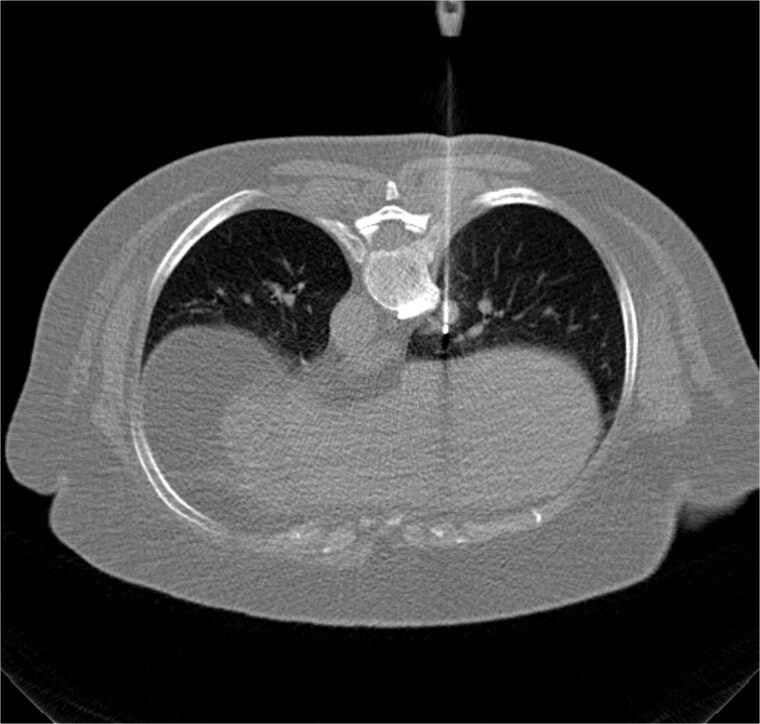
Axial non-contrast chest CT obtained during the procedure shows placement of a radiofrequency ablation probe within the right lower lobe pulmonary nodule appropriately positioned within the lesion.
